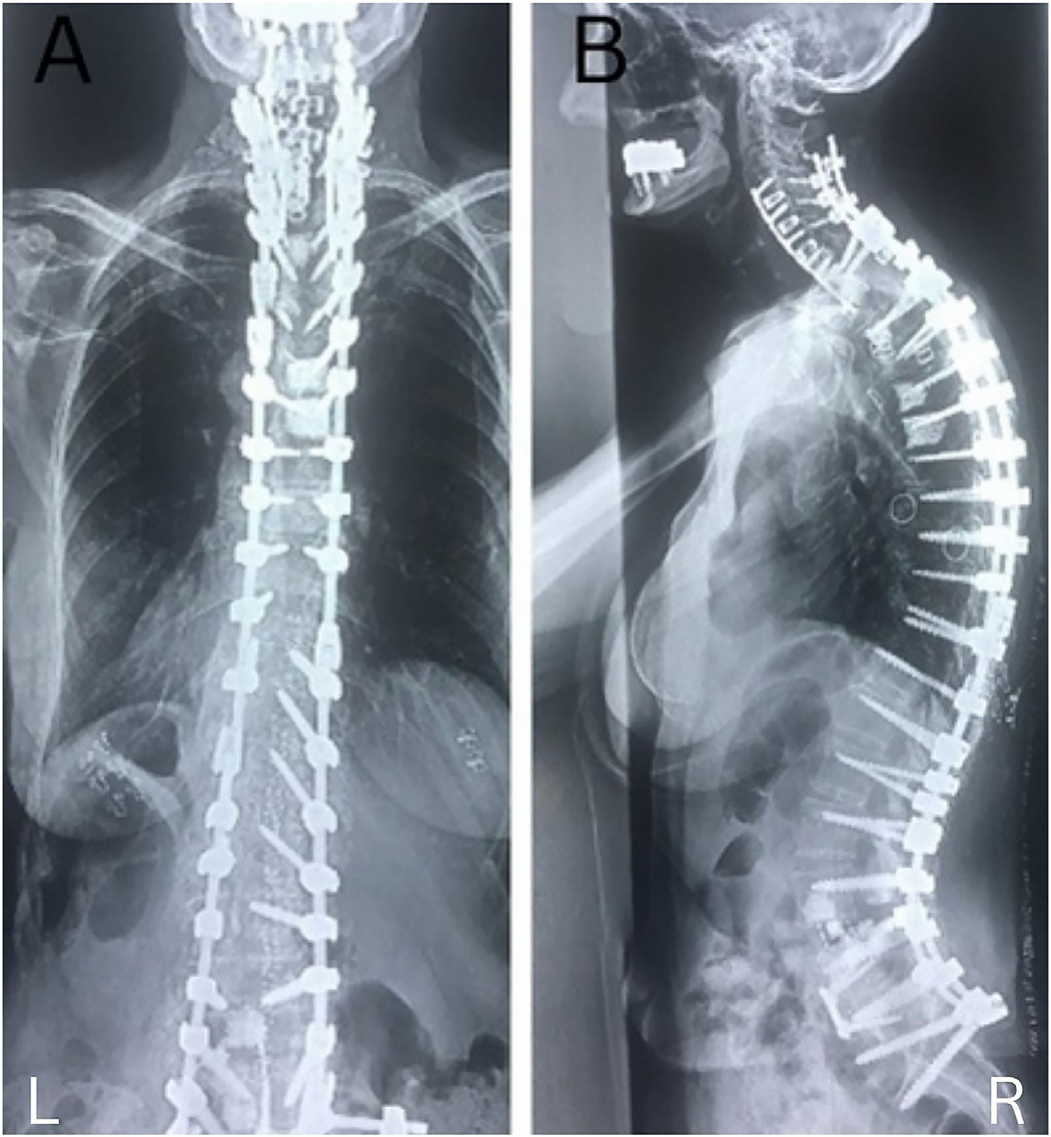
A. Anteroposterior radiographs demonstrating final postoperative construct. B. Lateral radiographs demonstrating final postoperative construct.
